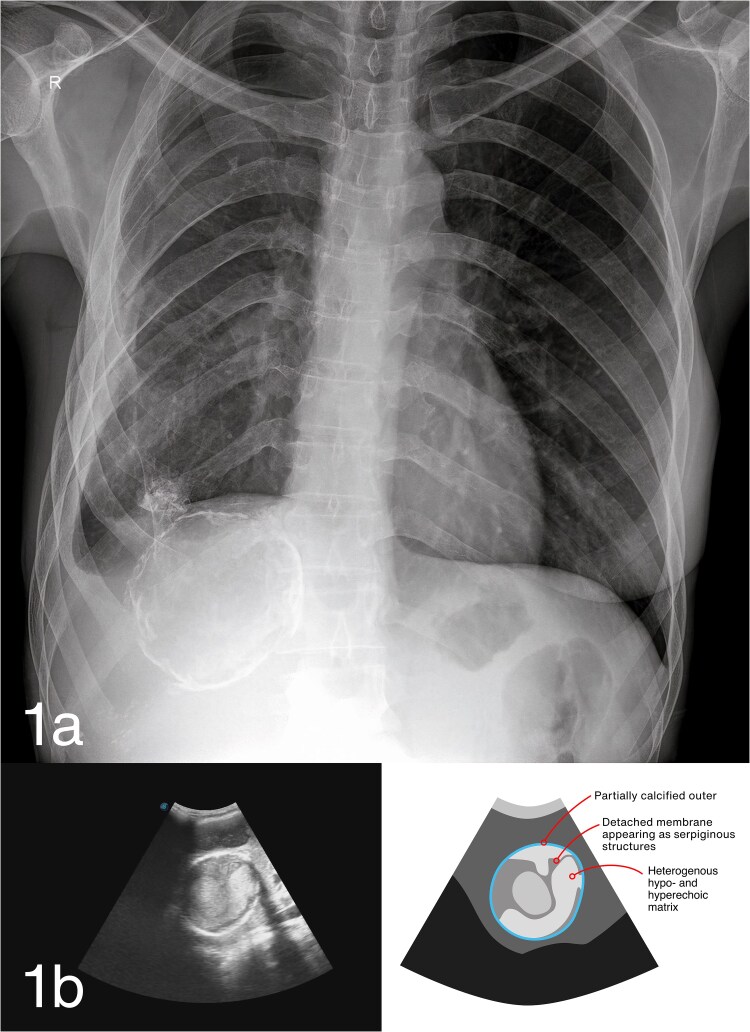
a) Chest X-ray showing a well-circumscribed opacity in the right lower lung and a ring-calcified lesion in the right upper abdominal quadrant; b) abdominal ultrasound showing hepatic hydatid cyst CE4 with internal non-homogeneous content, serpiginous structures (cerebral *gyri* sign), and partial calcification.
